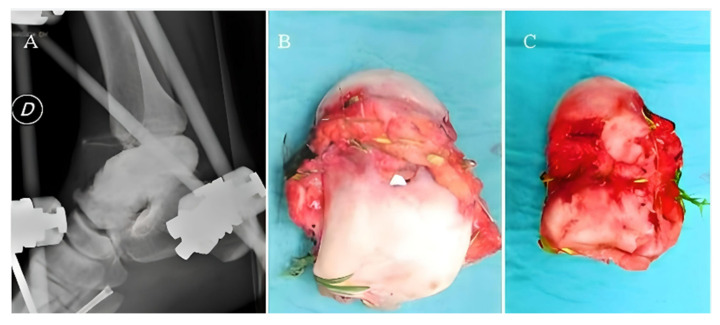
Latero-lateral ankle radiograph (A) shows the placement of the cement spacer and the ankle stabilized by external fixation. The talus as it appeared right after being collected from the site of the plane crush: a severe macroscopic contamination can be observed (B,C).
